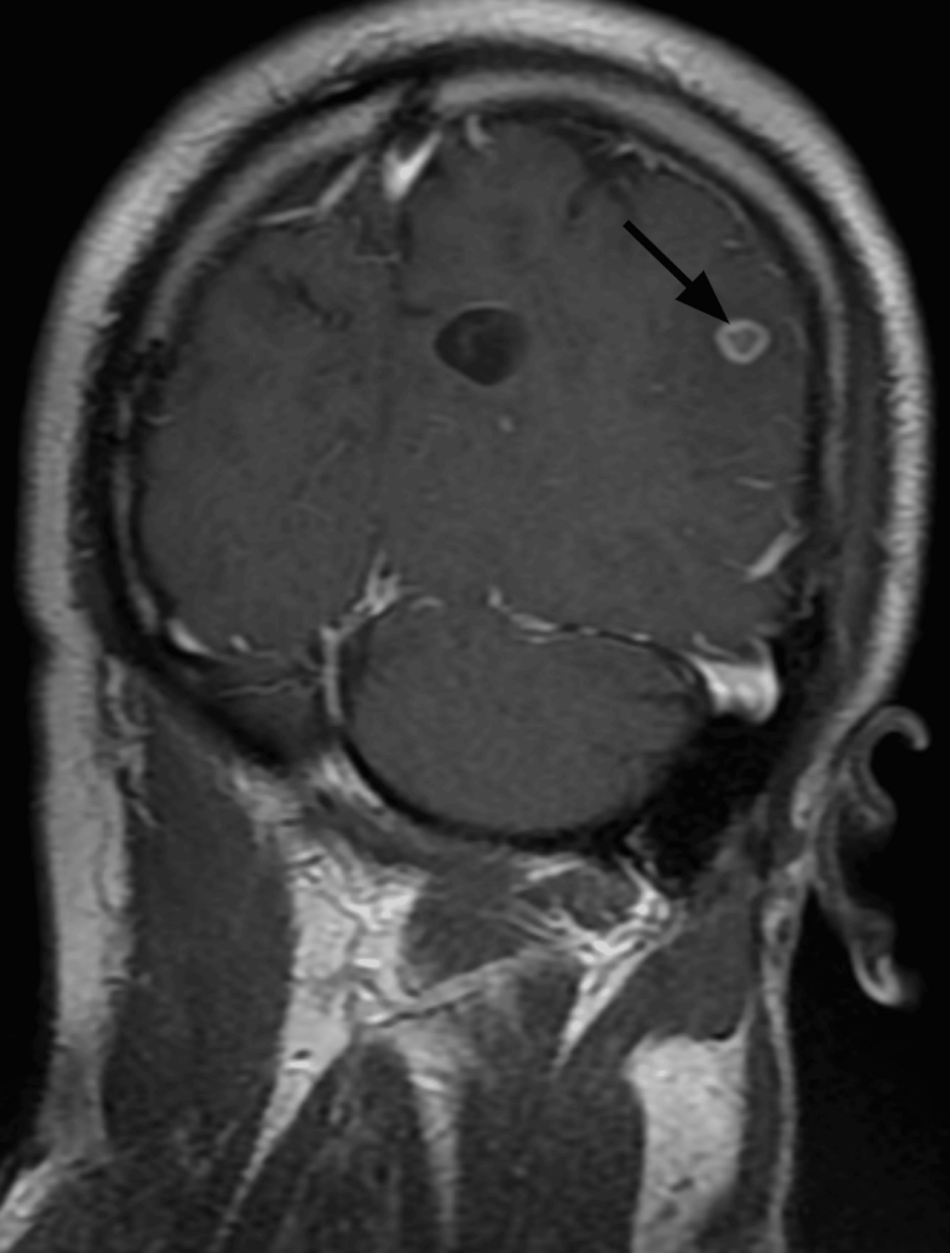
MRI of the brain in the coronal plane confirms the presence of a cystic lesion in the left parietal lobe
